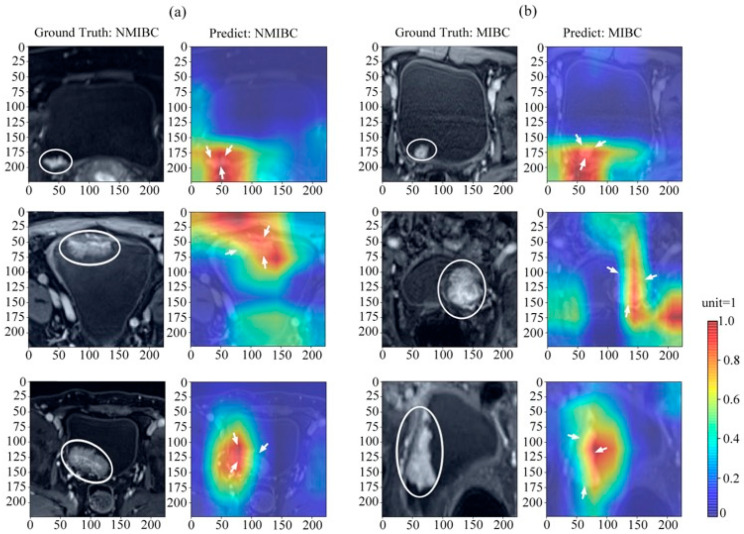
Spatiotemporal attention visualization for (a) NMIBC and (b) MIBC prediction using multi-temporal-phase DCE-MRI, and white elliptical circules and white arrows represent the tumor region in both CT images and the corresponding hot maps, respectively.
